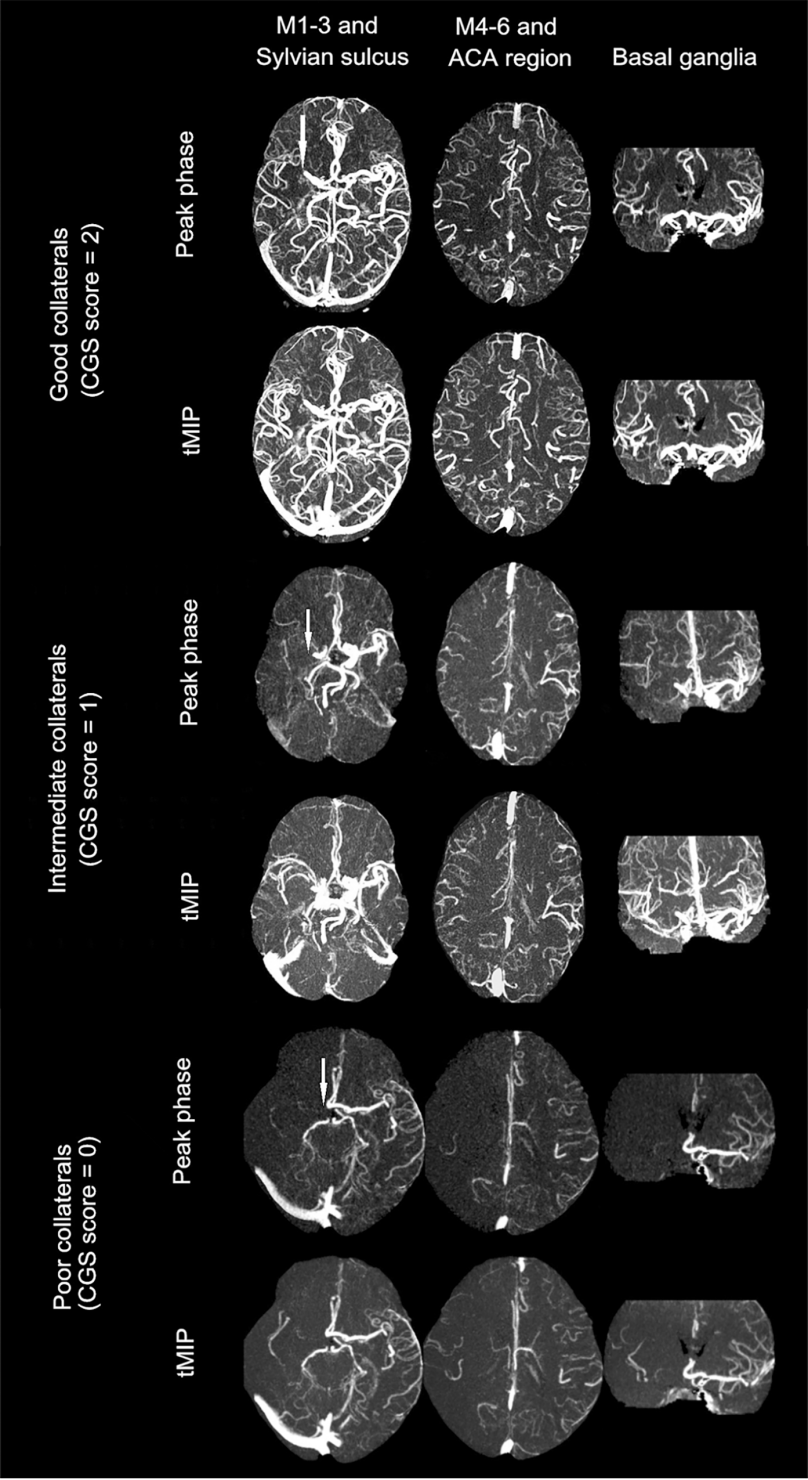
Collateral status on dynamic 4D CTA as measured by collateral grading scale (CGS). White arrow indicates the site of occlusion. Top two rows: a patient with a right MCA M1 segment occlusion and good collaterals as CGS score of 2: rLMC score on peak phase (rLMC-P) = 15, and rLMC score on tMIP (rLMC-M) = 18. Middle two rows: a patient with a right ICA occlusion and intermediate collaterals as CGS score of 1 (rLMC-P = 13 and rLMC-M = 15). Bottom two rows: a patient with a right ICA occlusion and poor collaterals as CGS score of 0 (rLMC-P = 3 and rLMC-M = 4).
